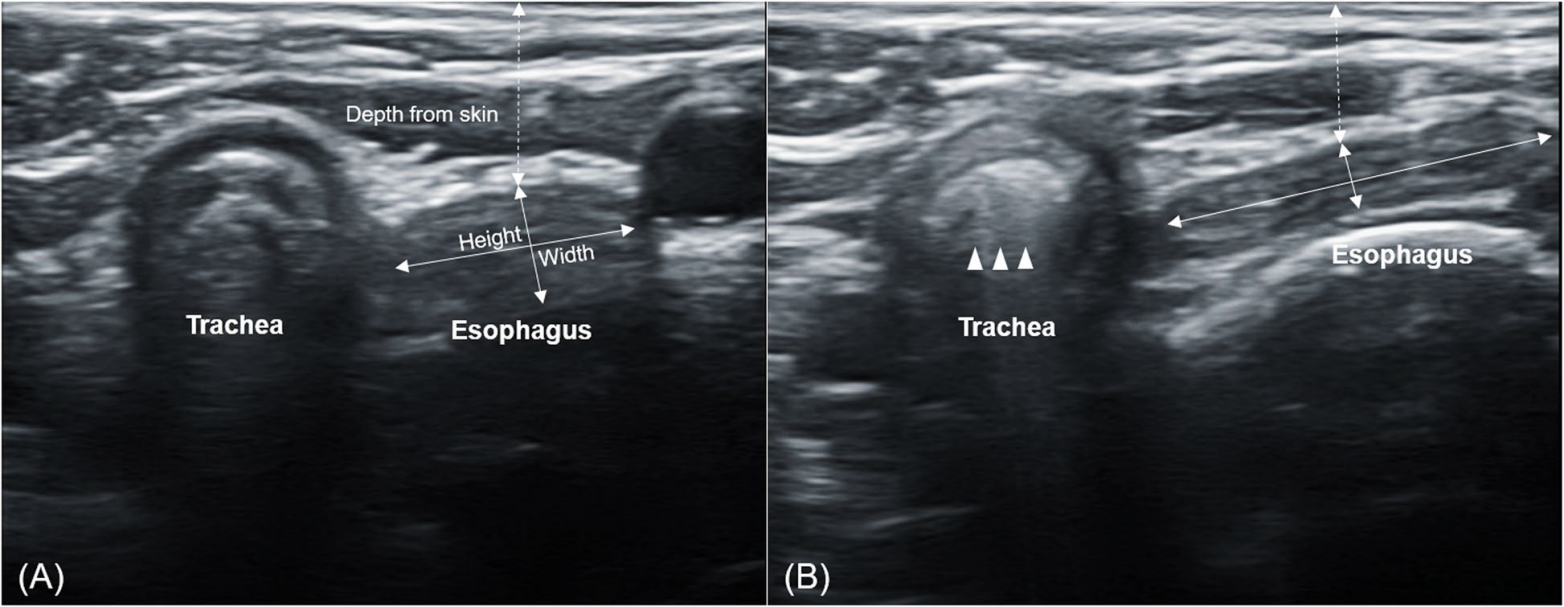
Before (A) and after (B) ultrasound-guided esophageal pressure application in a girl aged 14 months and weighing 12.2 kg. The arrows indicate tracheal insufflation during positive pressure ventilation
